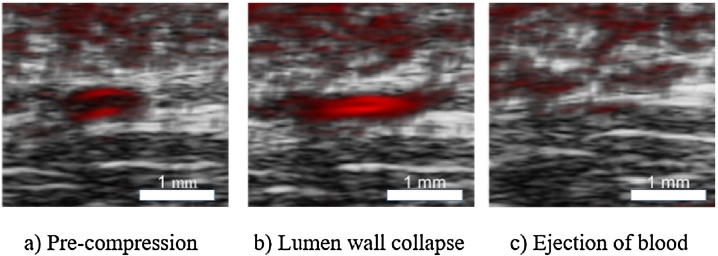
Collapse of a vessel tracked by photoacoustic–ultrasound dual imaging.
